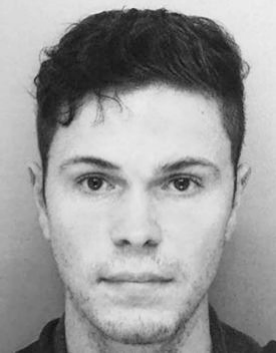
Tanguy Bois is a cardiology resident (4th year) at Rennes University Hospital, specializing in echocardiography. His fields of interest are cardiomyopathies and heart failure. He has carried out internships in specialized units in the fields of heart failure, heart transplantation, sports medicine, and intensive care unit.
